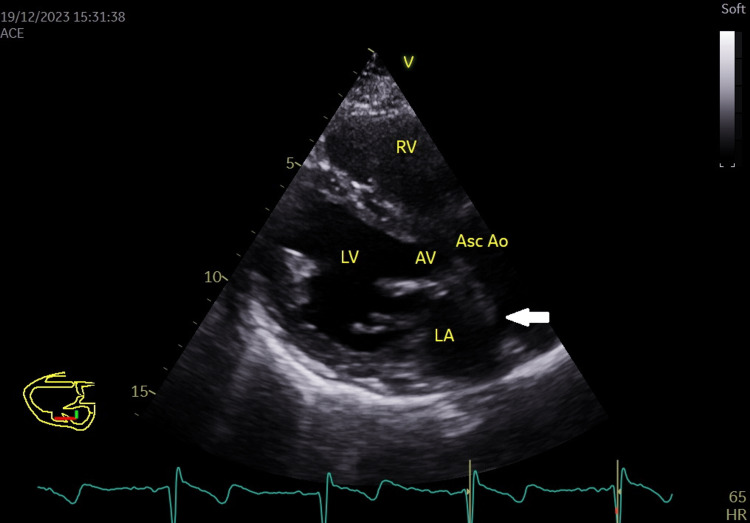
Parasternal long axis view from the left parasternal window showing the aortic mechanical valve with its reverberation (white arrow) in the ascending aorta and left atrium. Asc Ao: Ascending aorta; LA: Left atrium; LV: Left ventricle; RA: Right atrium; RV: Right ventricle.
